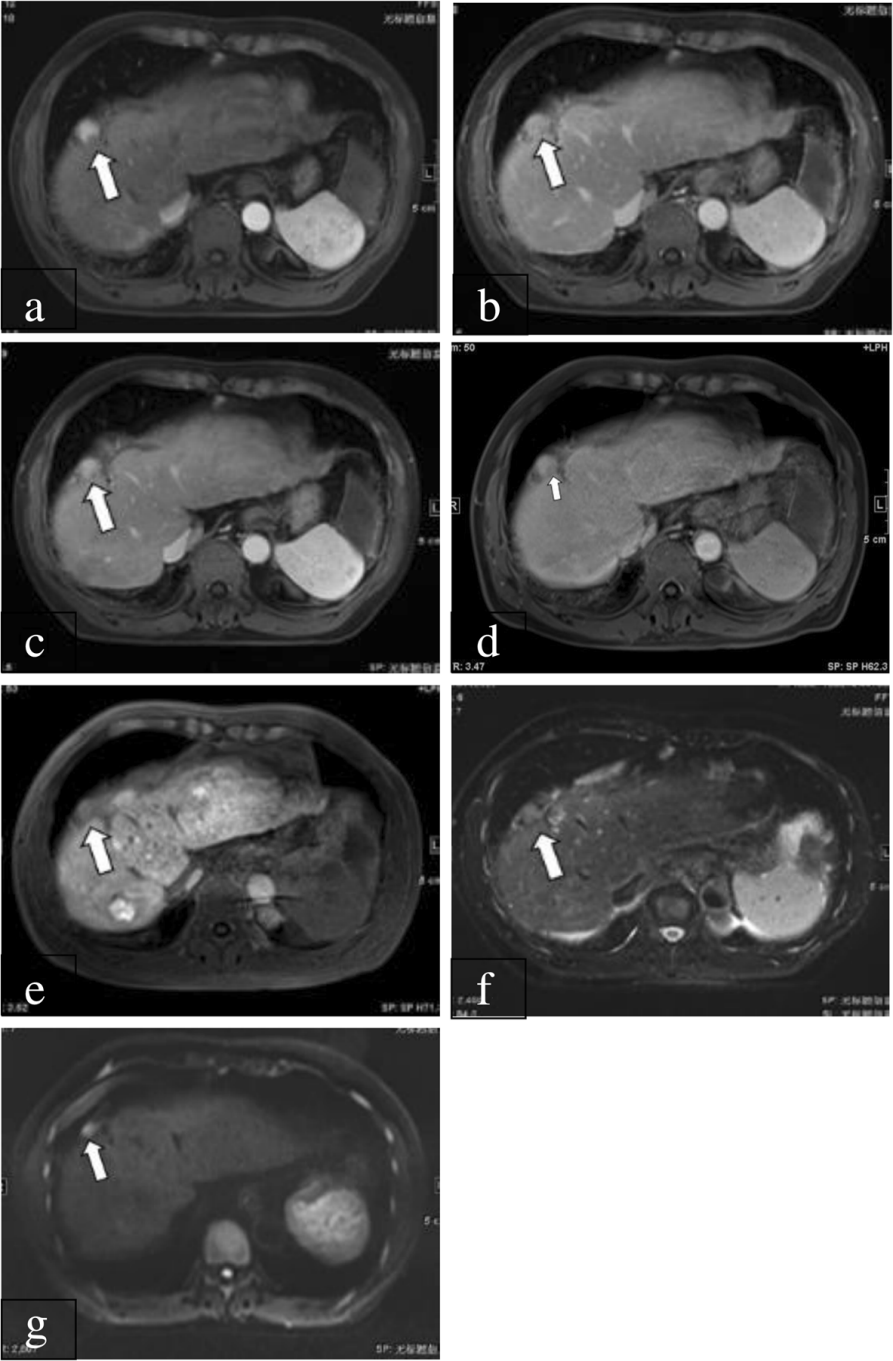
A 57-year-old male patient with HCC confirmed by surgery. a: On gadopentetate dimeglumine-enhanced 1.5-T MRI, there was a 20-mm nodule (arrow) showing arterial enhancement. b: During the PVP, the nodule (arrow) was isointense (no washout). c: During the delayed phase, the nodule (arrow) also showed no washout sign. d: Three days later, the patient underwent gadoxetic acid-enhanced 1.5-T MRI. During the PVP, the nodule (arrow) showed was isointense and hypointense. e: During the HBP, the nodule (arrow) was hypointense. f: On T2-weighted imaging, the nodule (arrow) was moderately hyperintense. g: On diffusion-weighted imaging, the nodule (arrow) showed restricted diffusion. Therefore, the preliminary LI-RADS category based on the major imaging features and considering the ancillary features was LR-4 using gadopentetate dimeglumine-enhanced 1.5-T MRI. After taking into account the sign on hepatobiliary phase, the LI-RADS category was upgraded to LR-5
